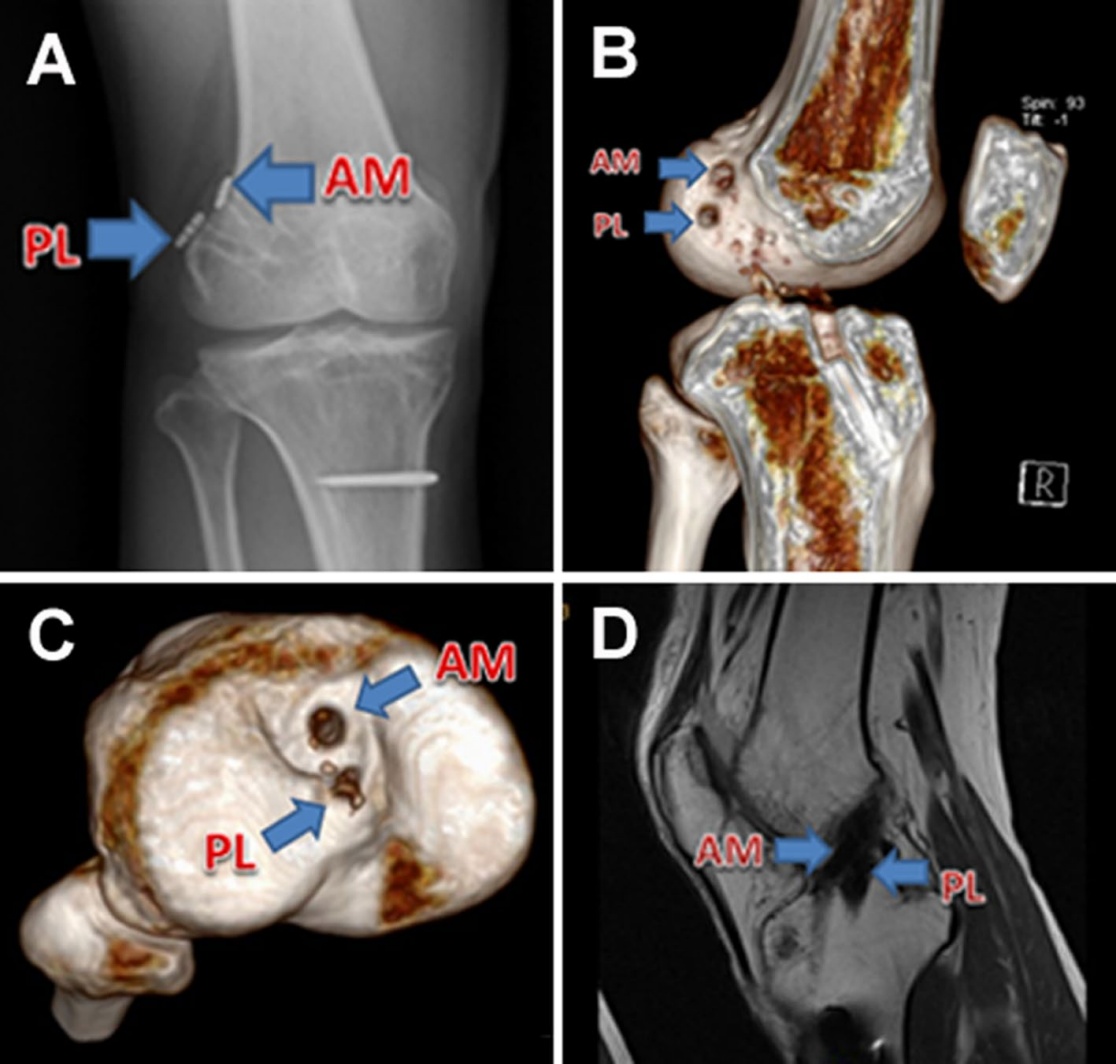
Postoperative radiological examination of double-bundle ACL individualized anatomical reconstruction after over 2 years. (A) X-ray: arrow shows the fixation of Endobuttons. (B) 3D CT: arrow shows the femoral socket. (C) 3D CT: arrow shows the tibial socket. (D) MRI: arrow shows the graft.
